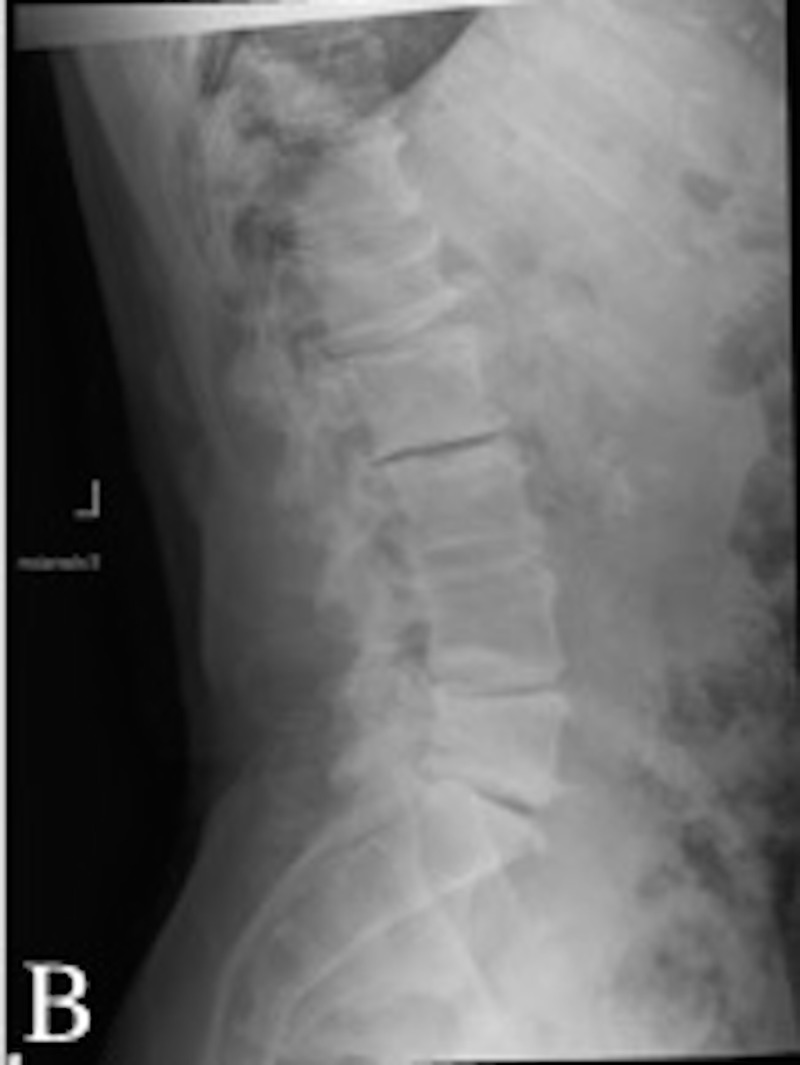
Pre-operative lateral X-rays. Pre-operative lateral X-rays degenerative scoliotic deformity, facet arthropathy, and neuroforaminal stenosis most severe in the lumbar spine.
